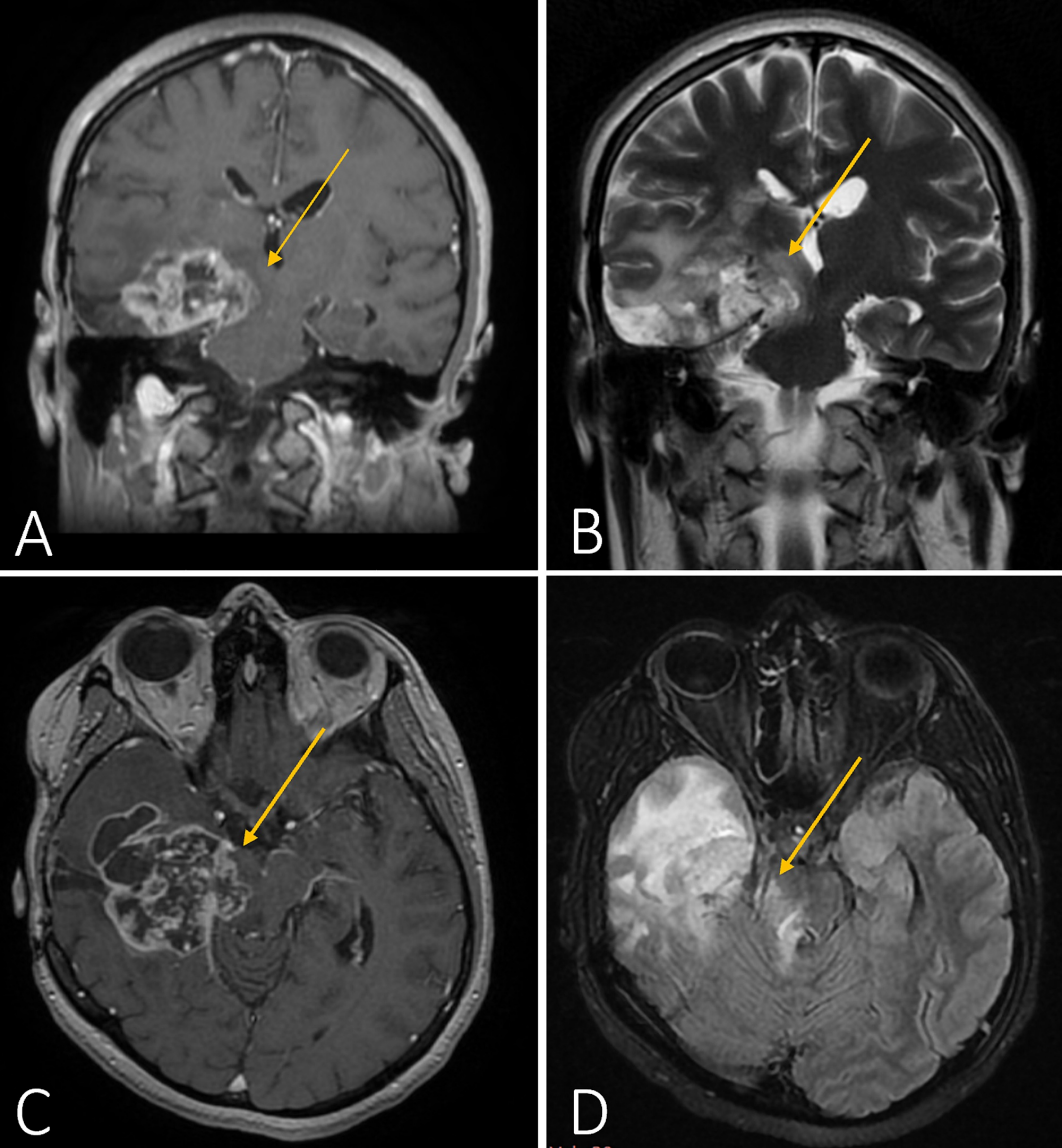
Cranial MRI prior to the third surgery Coronal (images A and B) and axial (images C and D) T1- and T2-weighted images, respectively, showcasing right-sided pontine extension of the tumor (yellow arrows) MRI: magnetic resonance imaging
